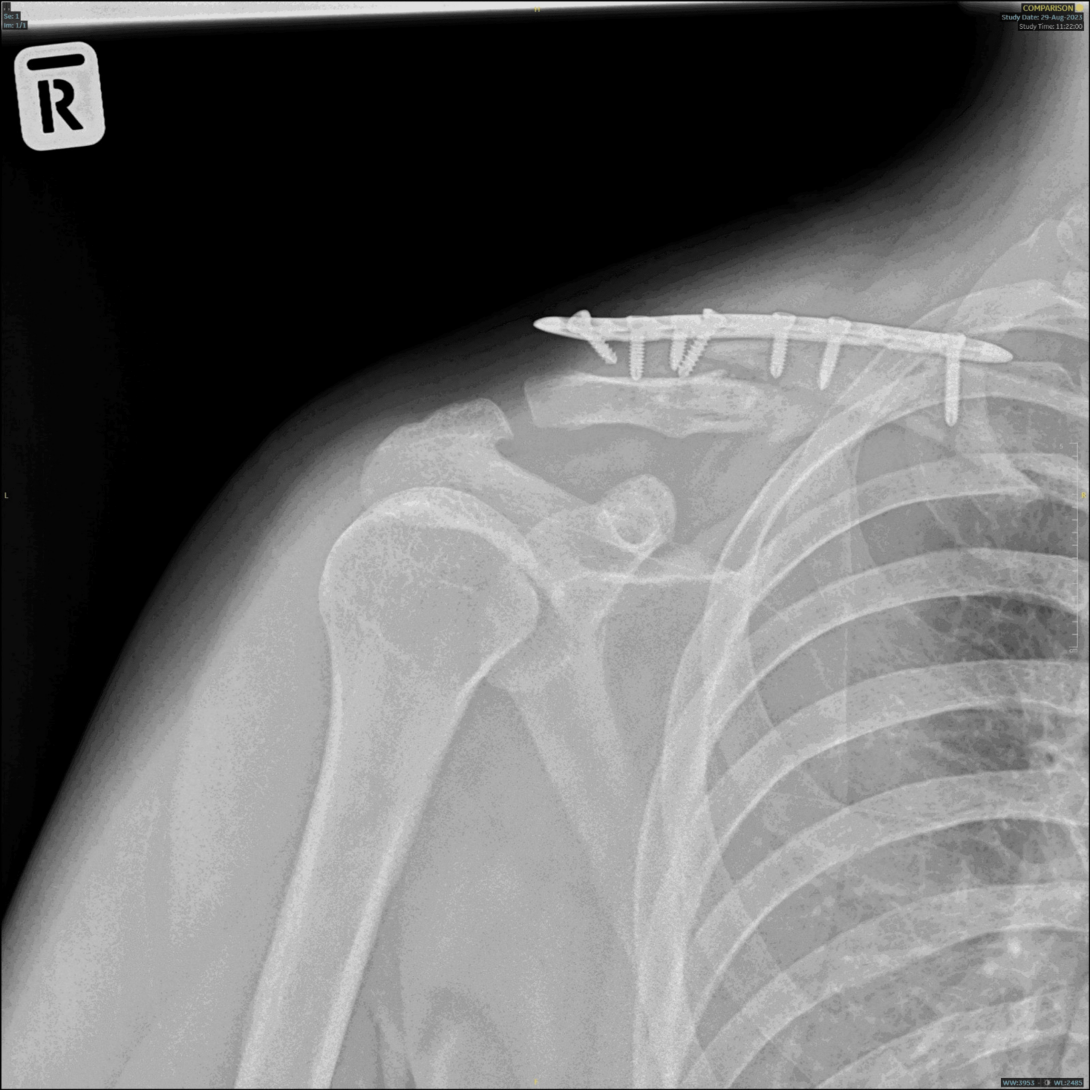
Plain radiograph four weeks after primary fixation. Screw cut out from distal clavicle with no callus formation.
